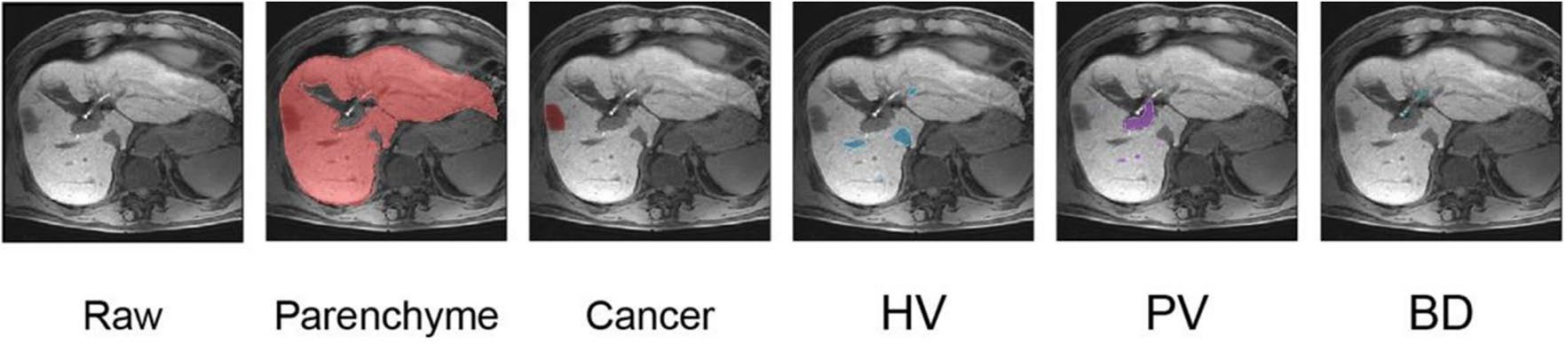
Manual segmentation of liver MRI for this study. Examples of slice-by-slice manual delineation of liver parenchyma, cancer, hepatic vein (HV), portal vein (PV), and bile duct (BD) by trained biomedical visualization artists are shown. They are confirmed by an abdominal radiologist and a hepatic surgeon.
